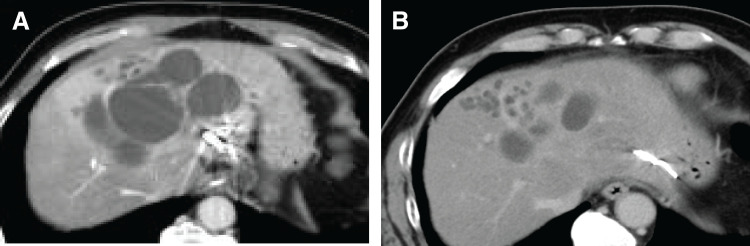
Enhanced CT after initiating GCS. (A) Taken 3 months later and (B) taken 6 months later. The tumor size decreased, and the remnant liver enlarged. GCS, gemcitabine/cisplatin/S-1
